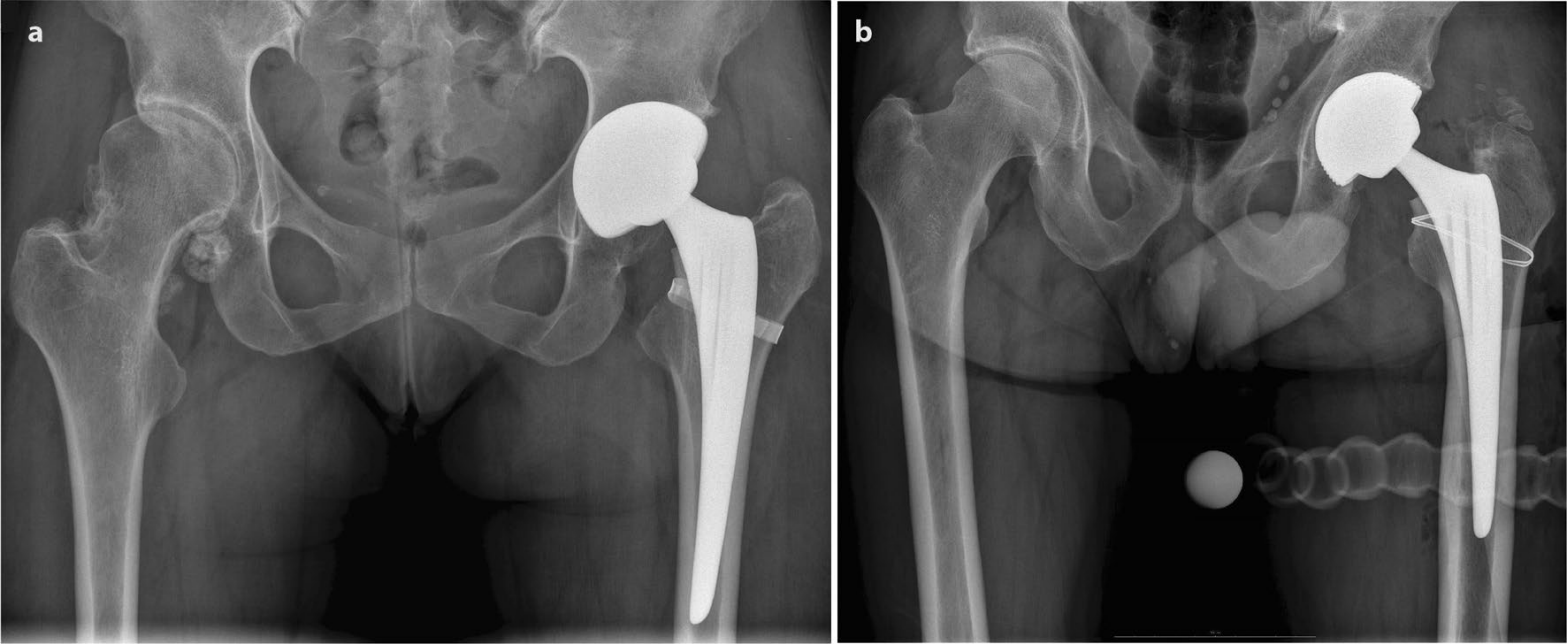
a Postoperative radiograph of intraoperative femoral fracture (IFF) on the right treated with titanium band. b Radiograph of patient with IFF treated with cerclage wire
